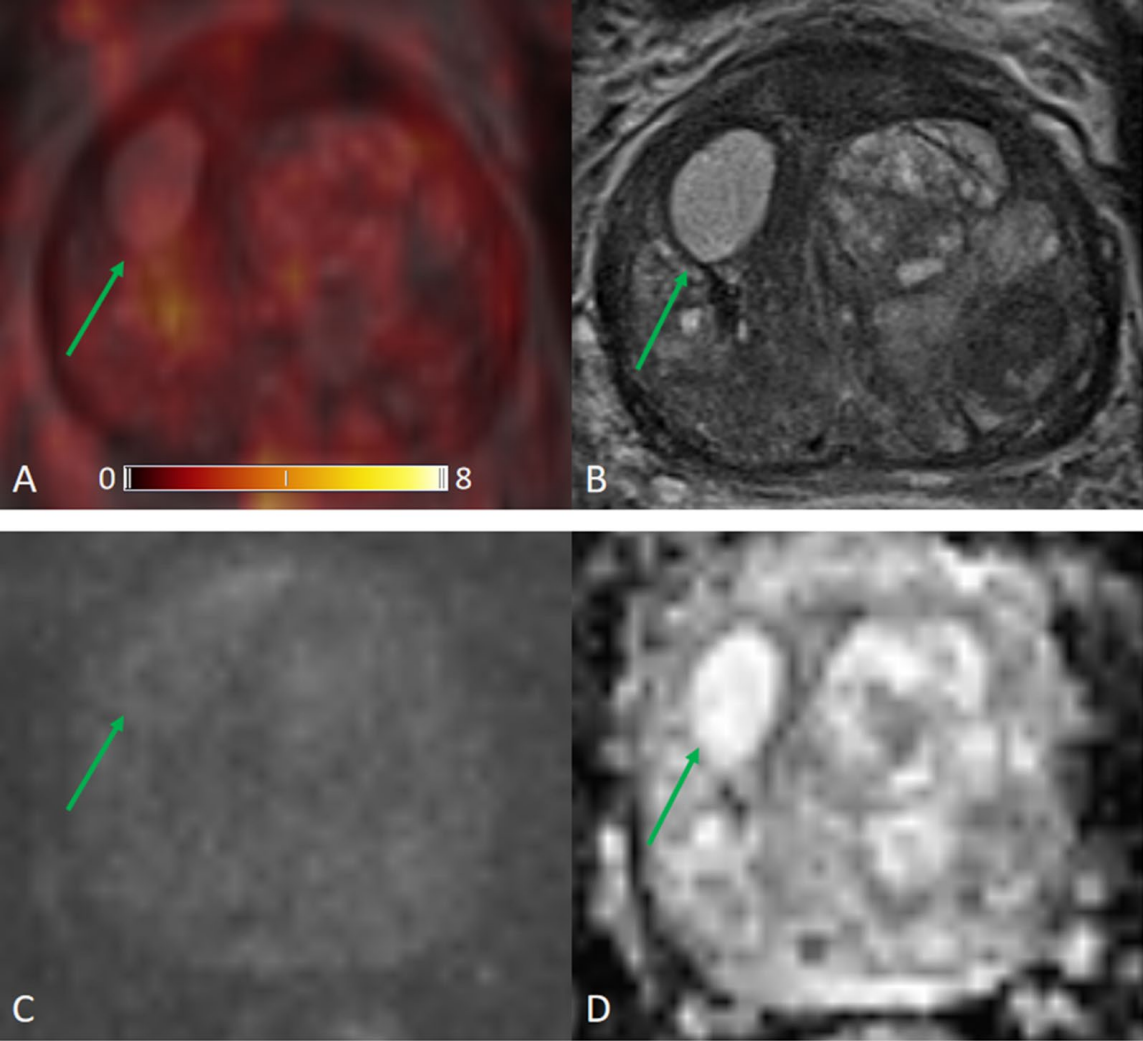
[^68^Ga]Ga-PSMA PET/MRI images obtained in a 72-year-old man, PSA 24.81 ng/mL. a [^68^Ga]Ga-PSMA PET/MRI; b T2WI; c DWI, *b* value 1000 s/mm^2^; d ADC. This example showed an MRI-negative lesions, a typical encapsulated nodule with unapparent radiotracer uptake in [^68^Ga]Ga-PSMA-11 PET. SUVmax 1.9, right TZ, background SUVmax 1.2, LBR 1.6
